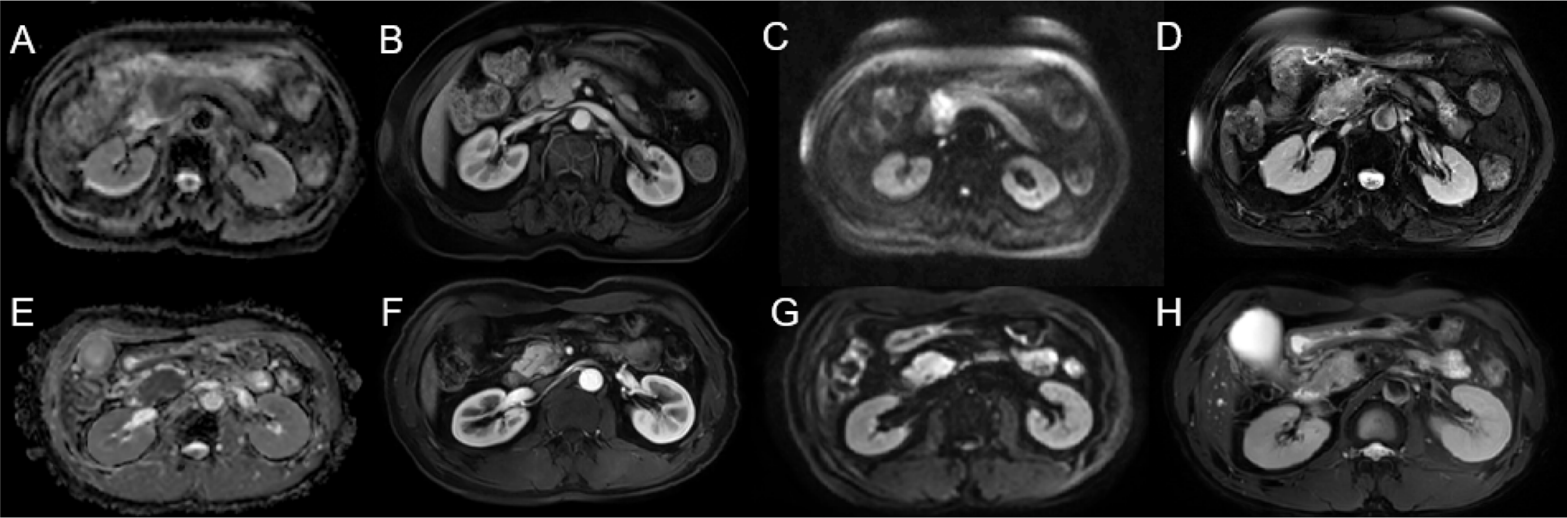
Two cases of multiparametric MRI images from pancreatic ductal adenocarcinoma patients with wild-TP53 (A–D) from a 78 year-old women and TP53 mutation (E–H) from a 53 year-old men. ADC map showed hypointense lesion (A, E). Slightly hypovascular lesion on late arterial phase (B, F). DWI sequence showing hyperintense lesion (C, G). T2WI showed slight hyperintensity of the pancreatic head mass (D, H).
